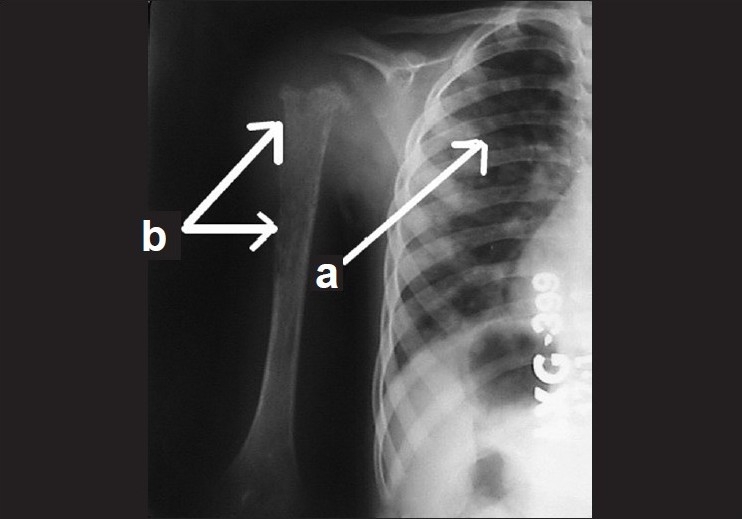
X-ray of the chest (a) and right shoulder joint (b) showing metastatic changes
